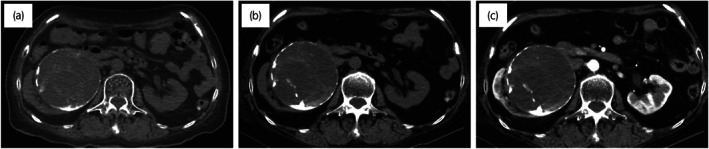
Abdominal CT. (a) Simple CT 1 year before surgery shows a 9 cm mass in the right kidney with calcification in the septum. (b) Simple CT and (c) contrast‐enhanced CT before surgery. CT shows an 11 × 10 cm mass in the right kidney, but the contrast effect is low.
